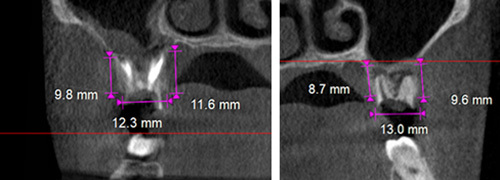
Shows the measurements of alveolar bone dimensions using CBCT scan before the surgical procedure (somatropin was applied in the socket of upper left molar).
